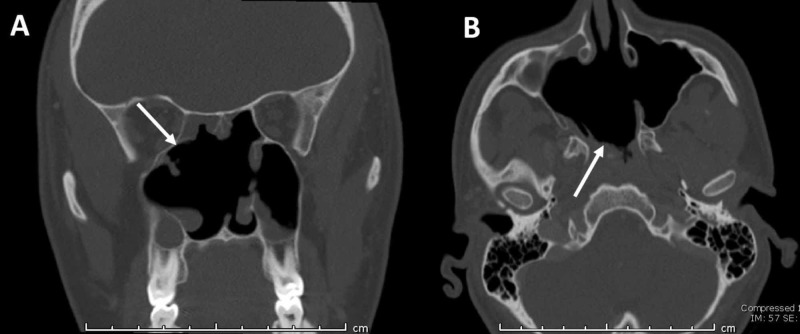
Postoperative CT images. (A) Coronal and (B) axial images showing complete tumor resection (arrows), bilateral maxillary antrostomies and sphenoethmoidectomies and posterior septectomy.
